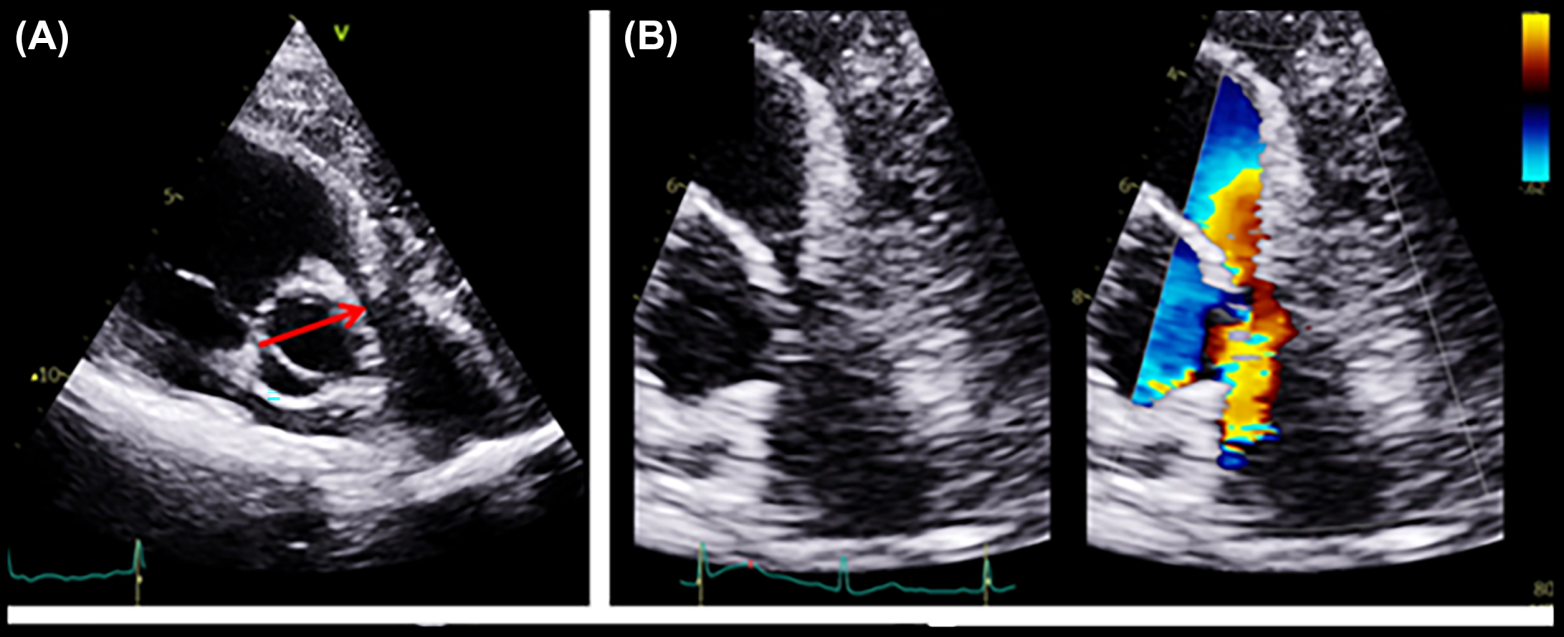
(A) Transthoracic echocardiography (TTE) parasternal short‐axis view at the great vessels level shows compression of the main pulmonary artery (mPA) (red arrow) by extra‐cardiac ill‐defined heterogeneous hyperechoic mass. (B) A focused view of the right ventricular outflow tract (RVOT) and mPA with colour Doppler shows aliasing in mPA suggestive of significant narrowing.
